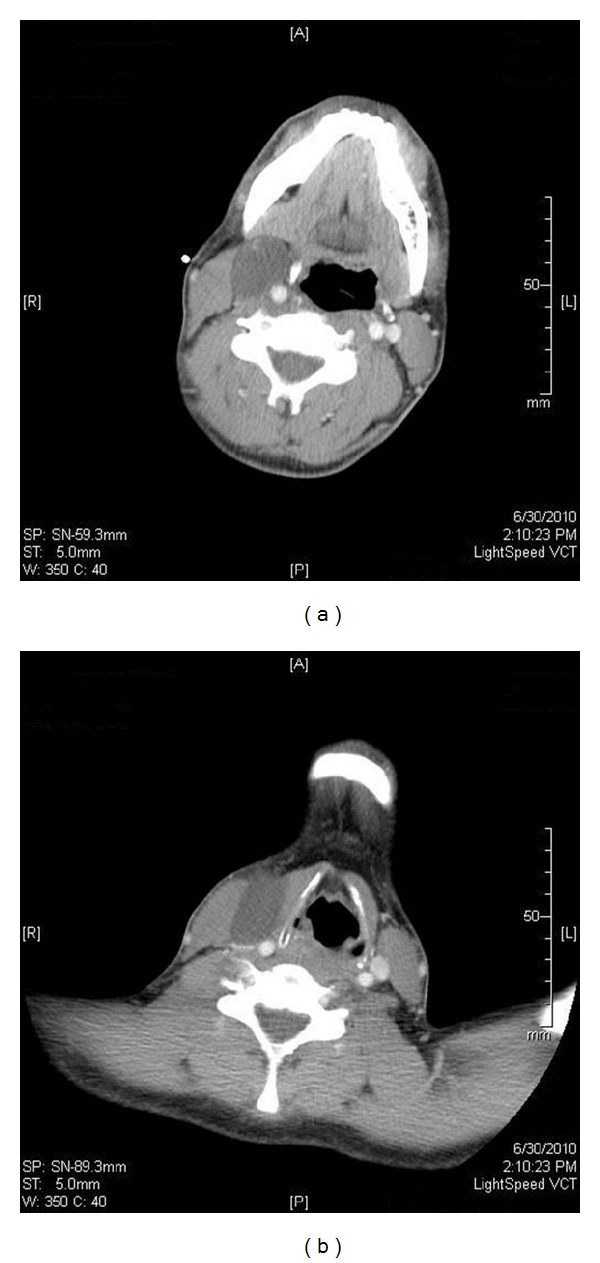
Axial CT Scan with contrast showing the cystic neck mass lateral to the great vessels.
